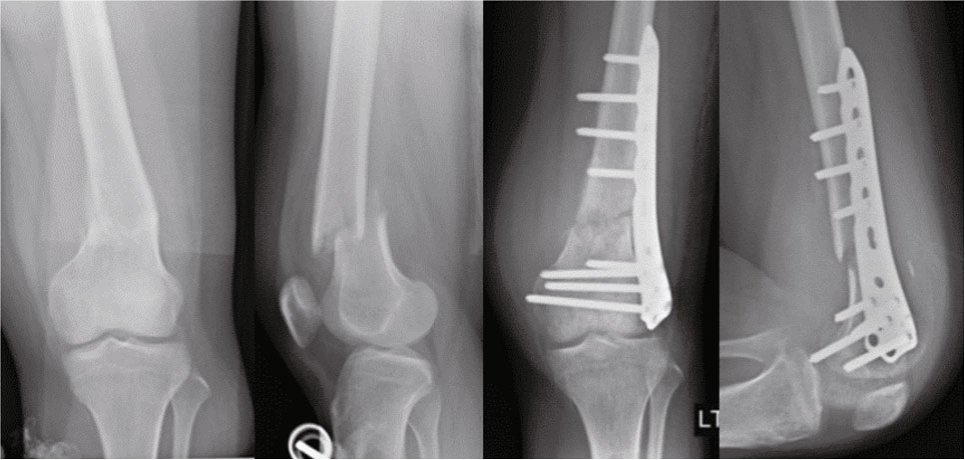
Pre- and postoperative X-ray of the unplanned fixation of the pathological fracture femur.
